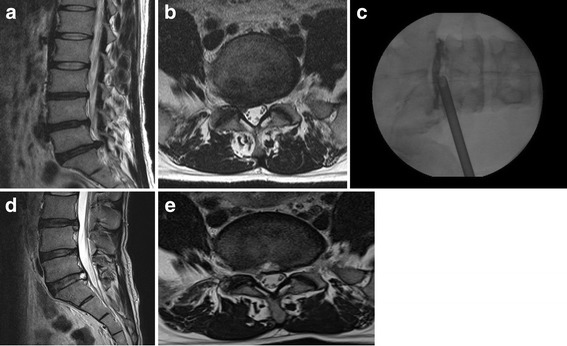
Four years later, MR images showing disc extrusion at the L5-S1 (a and b). After second PELD (c), and MR images showing complete decompression (d and e)
